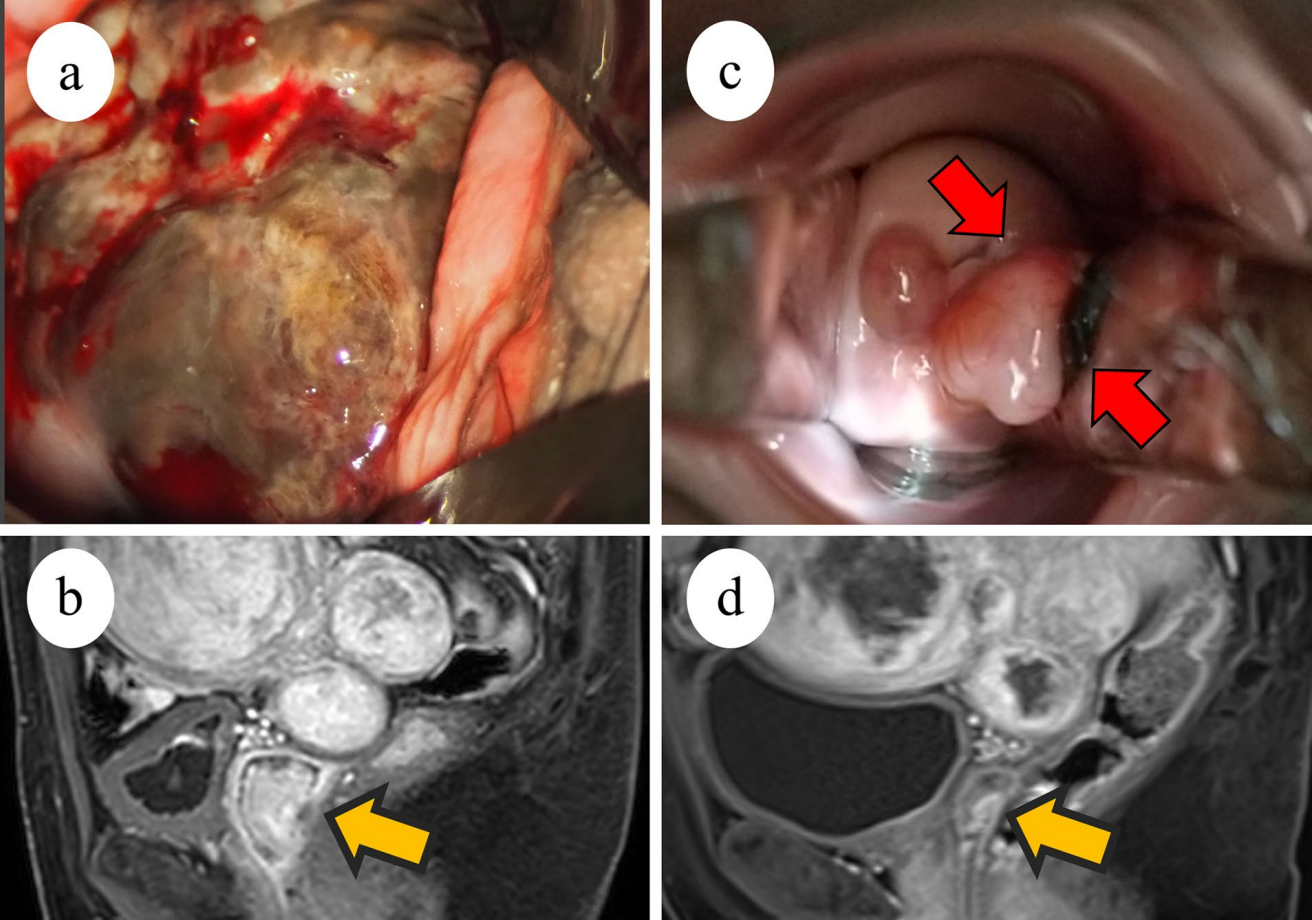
Clinical imaging of Case 2. a Colposcopy and b magnetic resonance imaging examination (yellow arrow, tumor) before combination therapy with ipilimumab and nivolumab. c Colposcopy (red arrows, tumor) and d magnetic resonance imaging (yellow arrow, tumor) after combination therapy with ipilimumab and nivolumab
